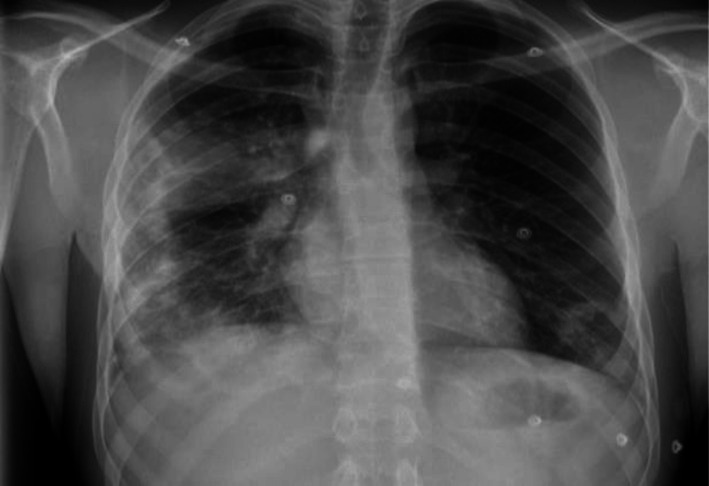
Initial chest radiograph showing bilateral patchy mid‐ and lower zone opacities, more prominent on the right. The diffuse, non‐lobar distribution is atypical for bacterial pneumonia and raised suspicion for a noninfective inflammatory process.
